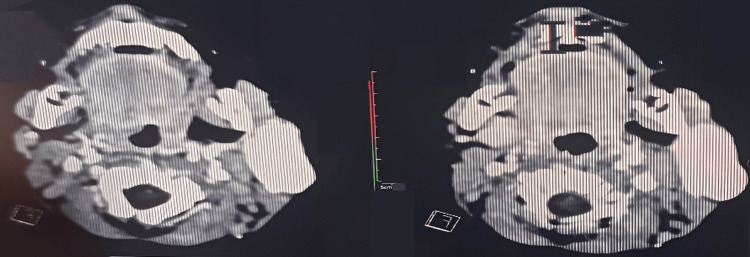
Cervical CT scan showing a hypervascular mass initially suggestive of a hemangioma.
